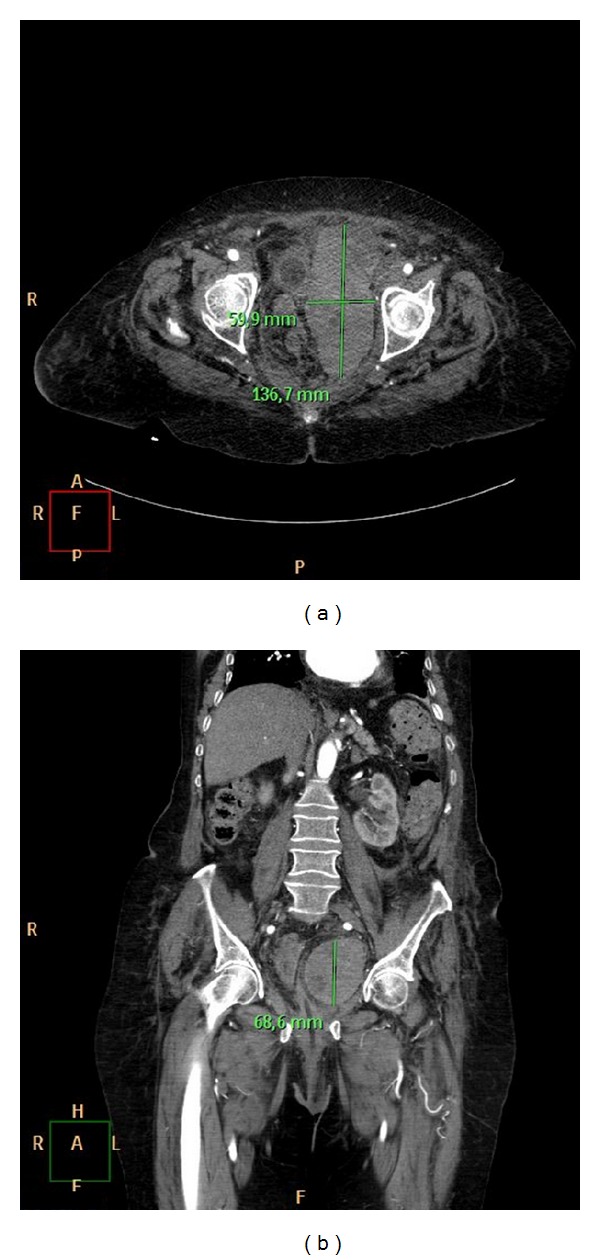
Axial (a) and coronal (b) CT of the pelvis demonstrating the size of pelvic haematoma.
